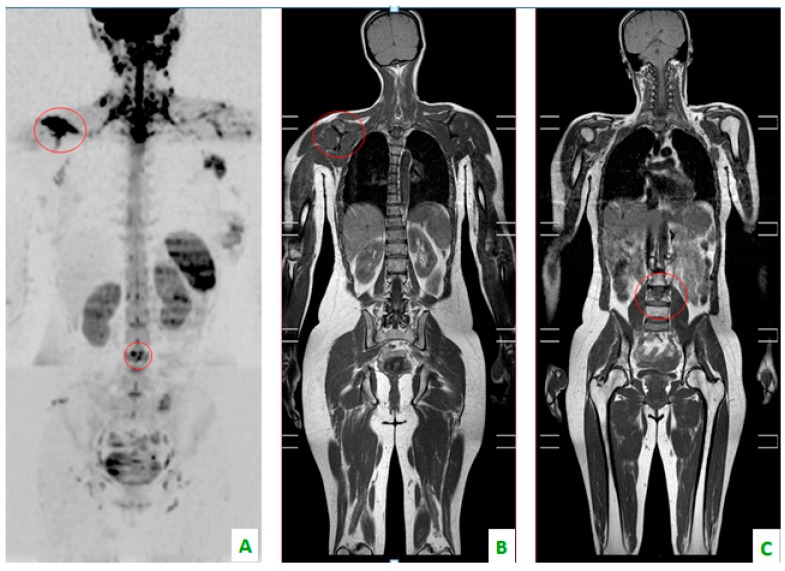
Bone localizations of breast cancer in the coronal plane: scapula and vertebral metastases (in the red circles). (A) DWI with a “positron emission tomography (PET)-like” view. The right scapula and one lumbar vertebra present an alteration of diffusivity. (B) T1 DIXON showing the hypointensity of the scapula and (C) lumbar soma.
